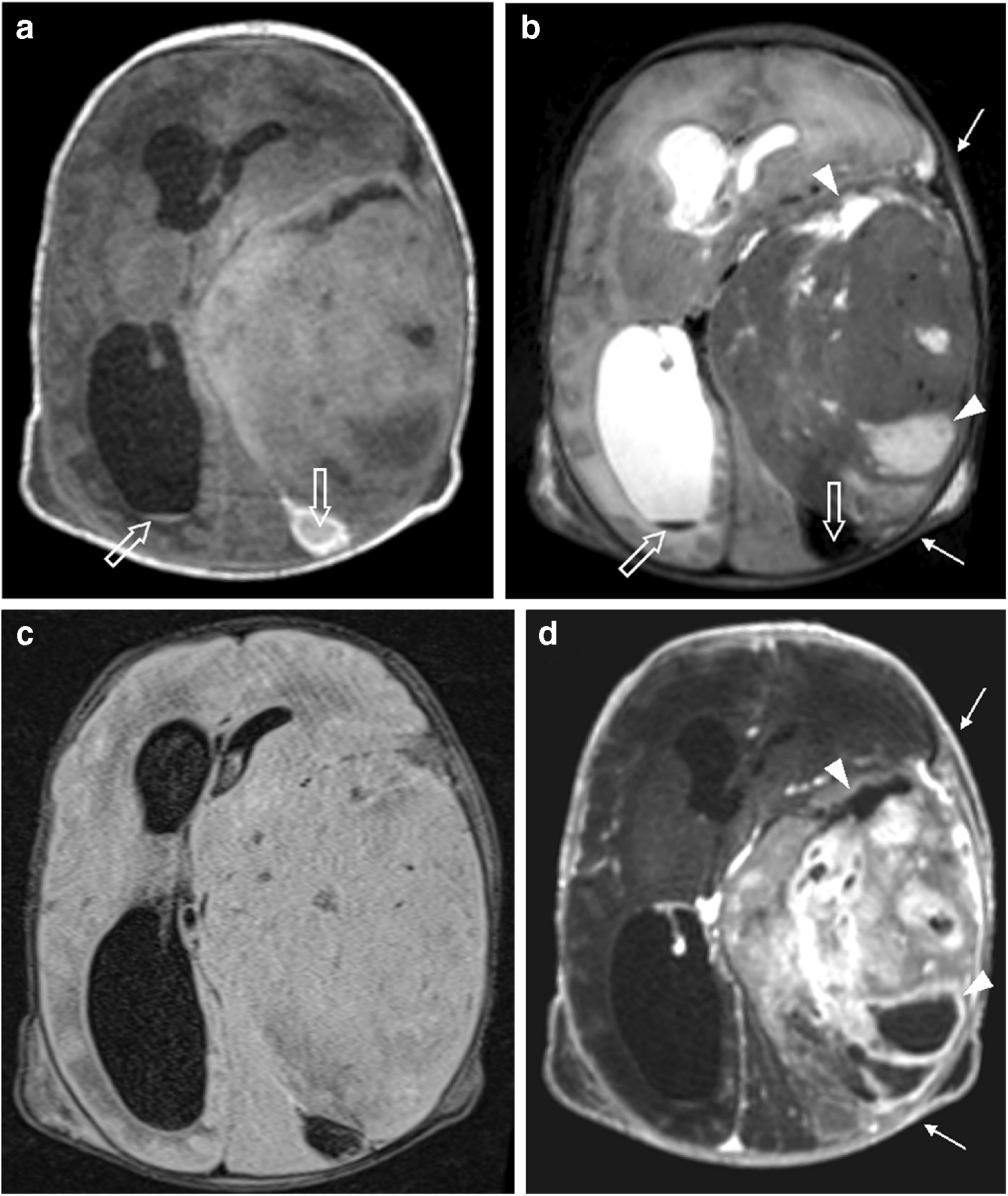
A 1-day-old girl with hypoxic-ischemic encephalopathy and a large left temporoparietal mass confirmed by surgery to be desmoplastic infantile ganglioglioma. a–d The mass is well circumscribed, peripherally (pial) based and mostly solid with heterogeneous signal showing iso- to mild hyperintensity on the pre-contrast axial T1 image (a) and predominant hypointensity (*solid arrows*) on the axial T2 image (b). Note a small amount of T1 hyperintense and T2 dark blood layering within the occipital horns (*open arrows*). Heterogeneously enhancing mass (*arrows*) and multiple internal non-enhancing cystic components (*arrowheads*) are poorly seen on the axial fluid-attenuated inversion recovery (FLAIR) image (c) but are depicted on T2 and clearly seen on the post-contrast axial T1 image (d). There is moderate mass effect and a rightward shift of midline structures causing near effacement of the left lateral ventricle and trapping of the right lateral ventricle but not significant surrounding edema. The mass and its extent are better delineated on the post-contrast T1 image (d) than on the pre-contrast T1 image (a)
